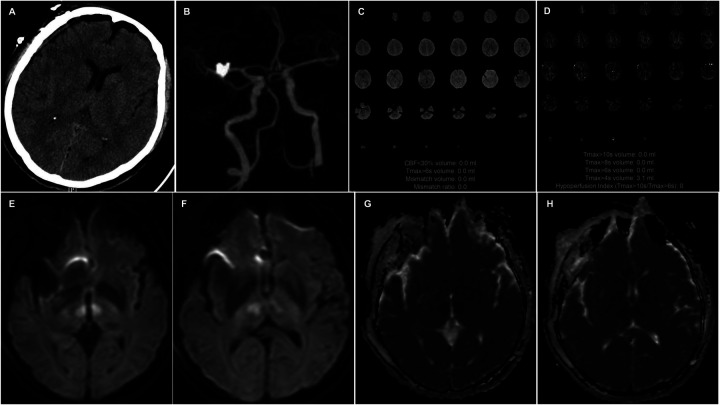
A noncontrast head CT (A) scan reveals no acute hemorrhage or infarctions. CTA (B) shows no large vessel occlusion, and CTP (C,D) indicates no definitive core infarction or hypoperfusion zones. Axial DWI (E,F) images show high signal intensity in the bilateral paramedian thalami, with corresponding decreased apparent diffusion coefficient (ADC) values (G,H).
